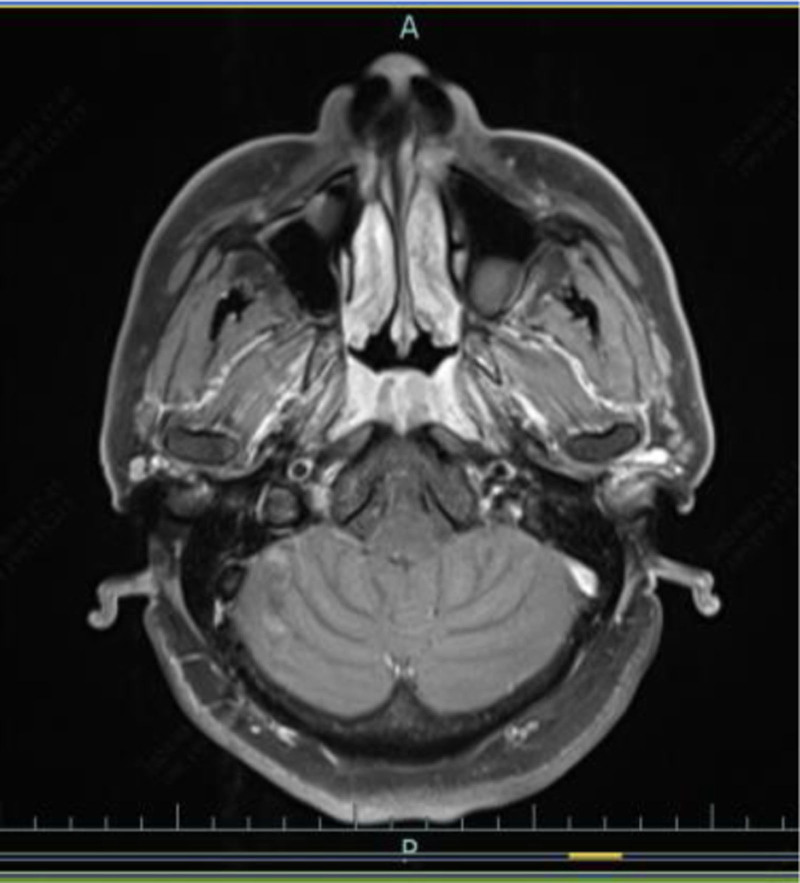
Nasopharyngeal MRI: no post-contrast enhancement is seen in the lesions. MRI = magnetic resonance imaging.
